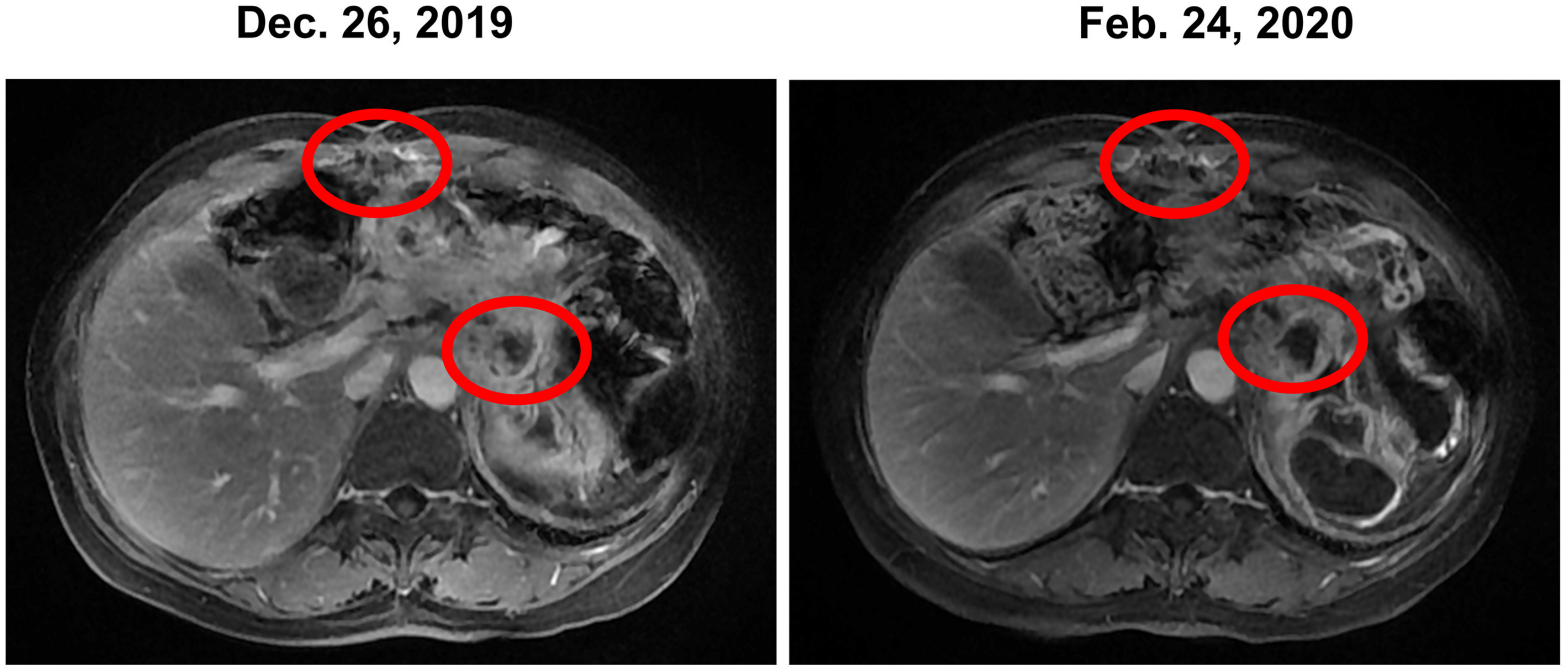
Stable disease was observed during the treatment of trametinib combined with hydroxychloroquine based on MRI. MRI, magnetic resonance images.
